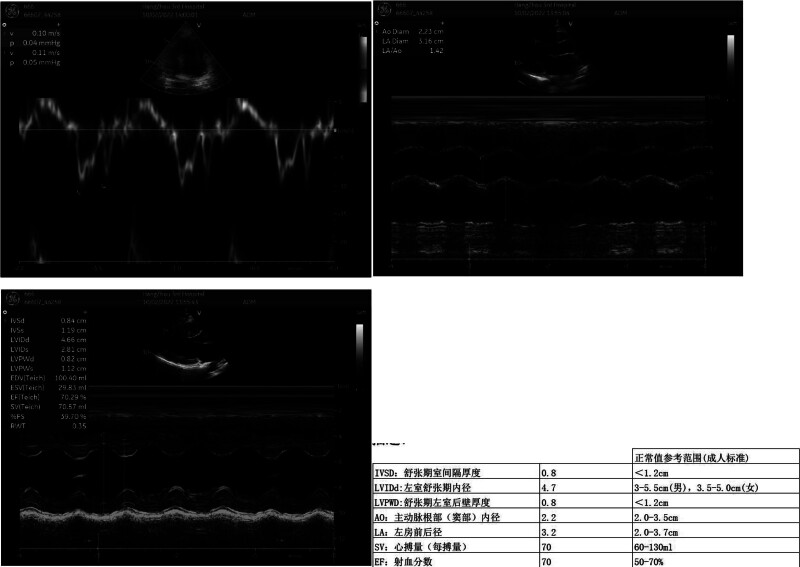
Echocardiography report during recovery.
